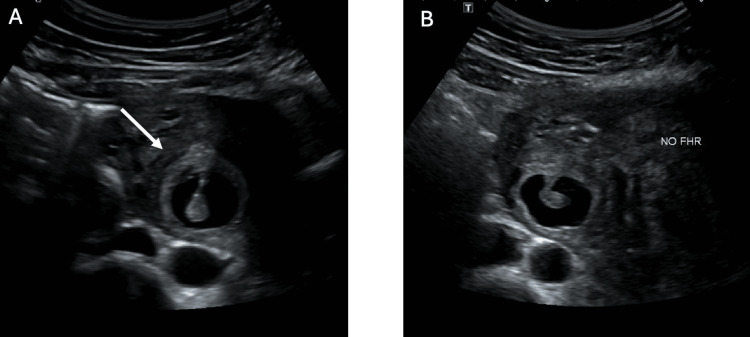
Ultrasound-guided administration of potassium chloride (KCl) (A) Ultrasound demonstrating disruption of the gestational sac and injection of the KCl. (B) Ultrasound post-administration of KCl demonstrating subsequent asystole in the cornual ectopic pregnancy.
